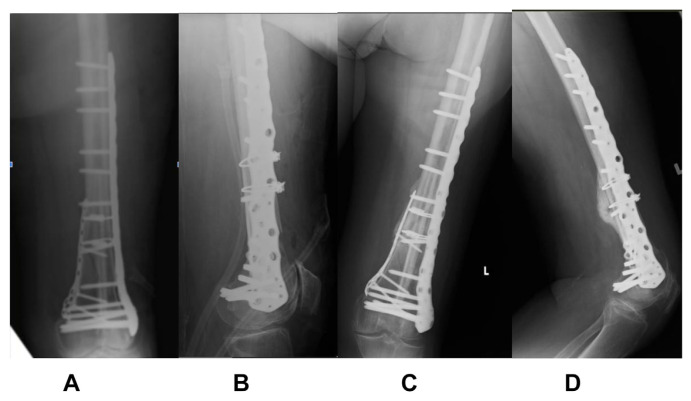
(A and B) Immediate postoperative radiographs. (C and D) Radiographs at 6 months after surgery.
